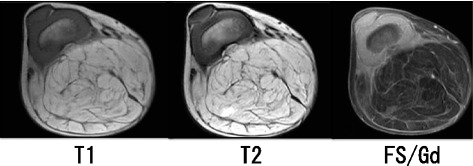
Magnetic resonance findings. The mass appeared hyperintense on T1-weighted (*left*) and T2-weighted images (*middle*) but not on fat-saturated T2-weighted images. Numerous internal fibrous partitions were visible on gadolinium-enhanced fat-saturated images (*right*). *FS* fat-saturated, *Gd* gadolinium-enhanced
